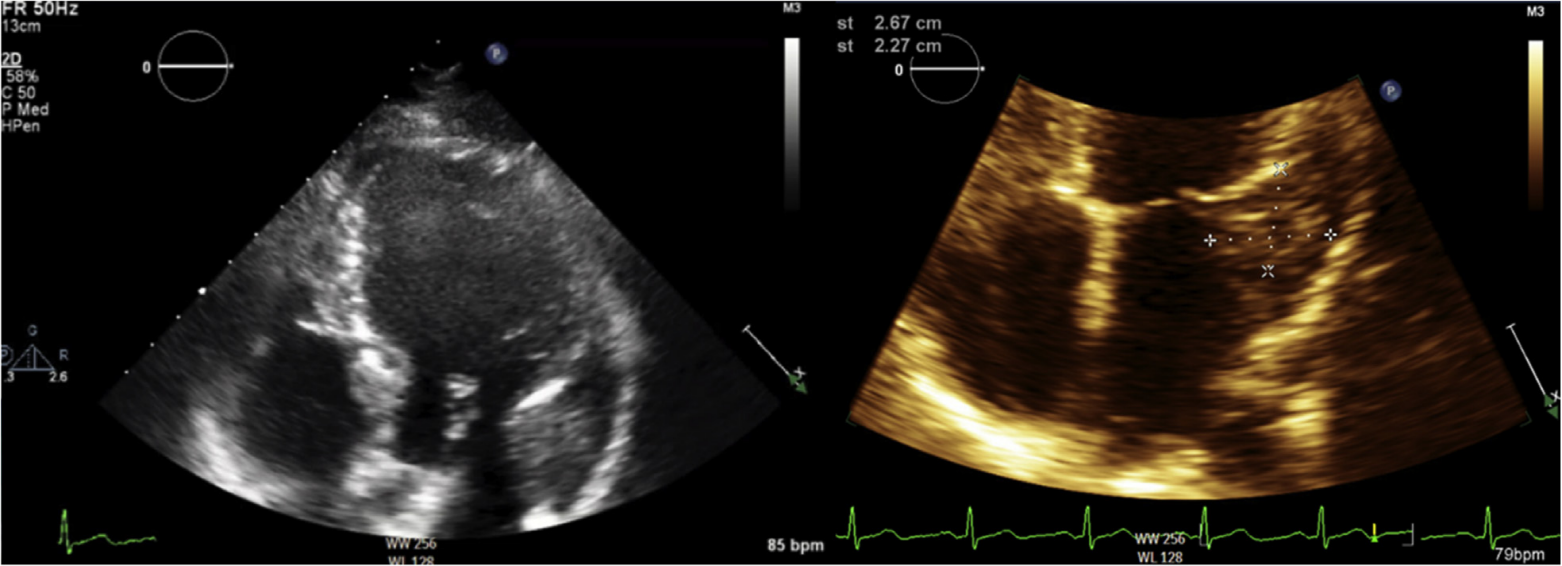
Transthoracic Echocardiography With the Mass on the Lateral Left Atrial Wall
